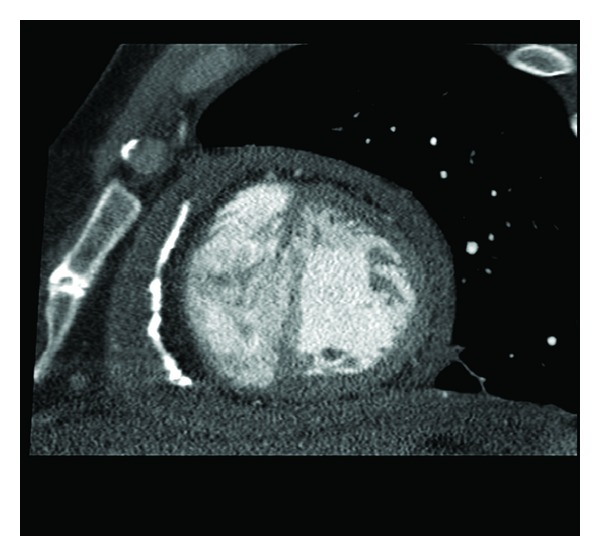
CT image indicating pericardial thickening.
